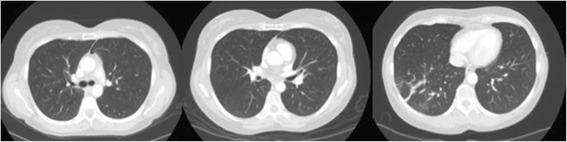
Computed tomography scans at levels of the mid-carina (branch point of the bronchi), mid-pulmonary artery, and lung base
